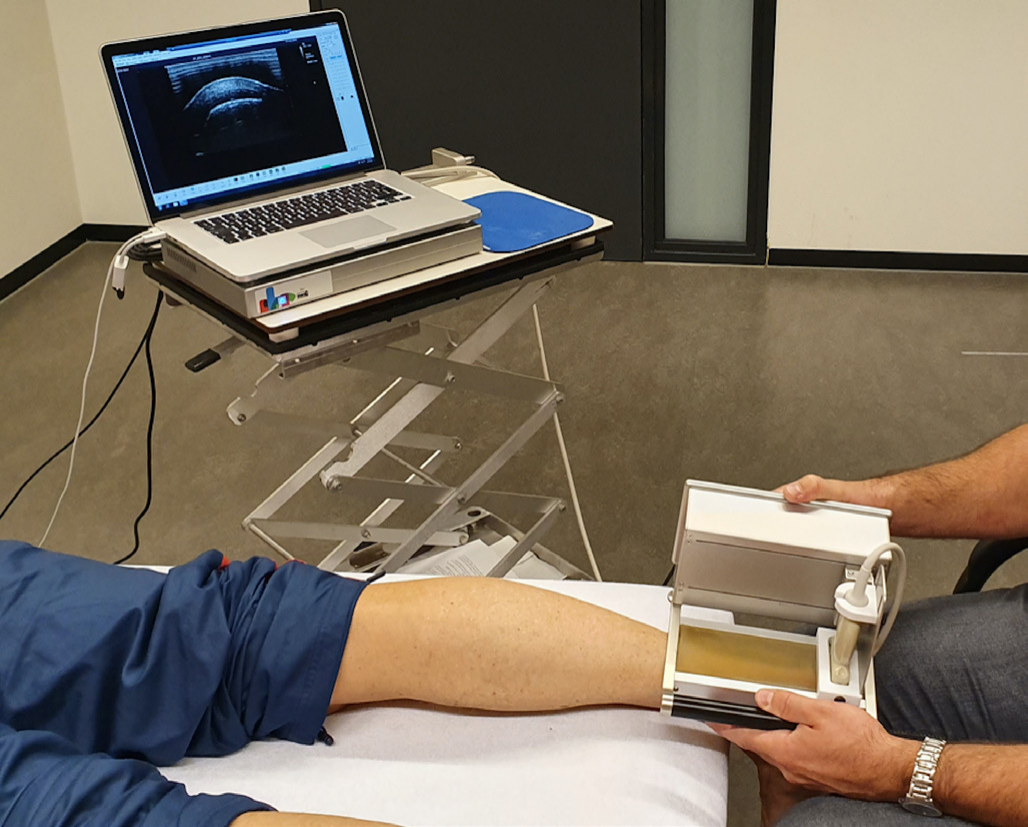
Ultrasound tissue characterisation scanning of the Achilles tendon with the patient in a prone position.
